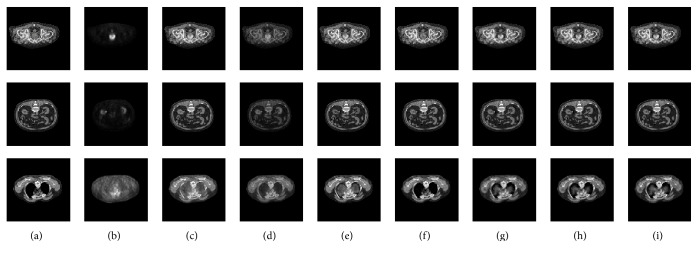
Three examples of the fusion results obtained by different methods. (a) CT images. (b) PET images. The (c)–(i) columns are fusion results produced by (c) DWT, (d) NSCT, (e) NSCT_PCNN_1, (f) NSCT_SF_PCNN, (g) NSCT_PCNN_2, (h) NSCT_PCNN_3, and (i) the proposed framework, respectively.
